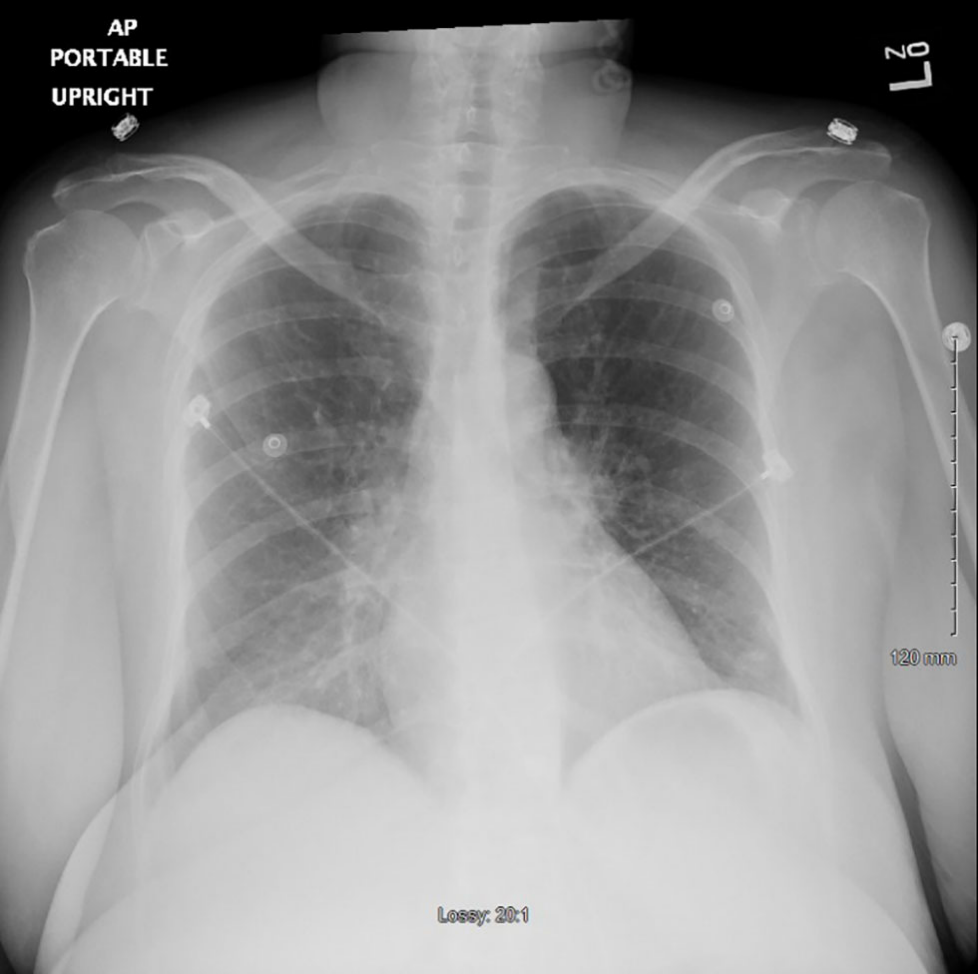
Chest x-ray 48 h after stopping clozapine (the second time) showing markedly improved lungs fields now almost completely clear.
